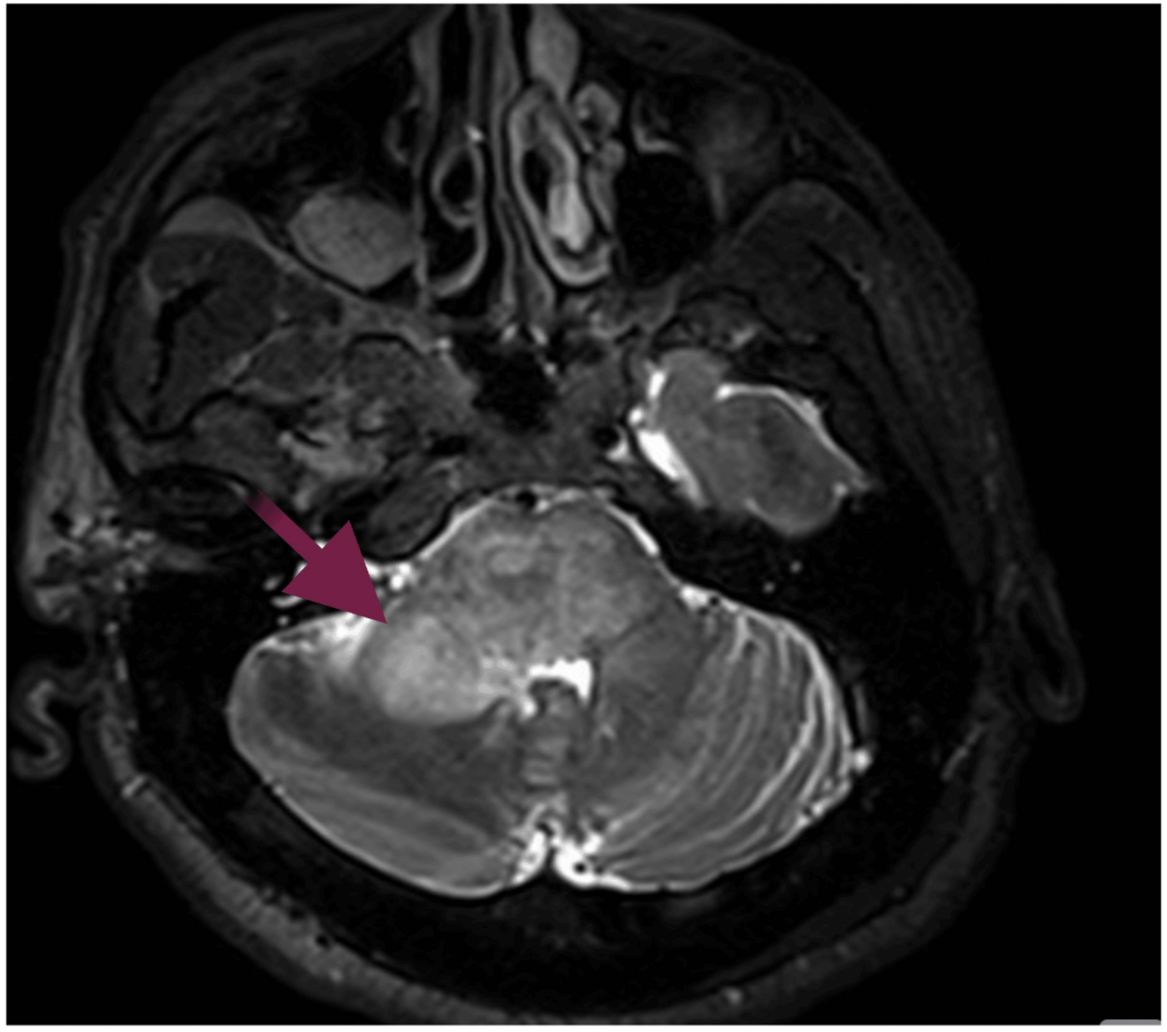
MRI of the brain MRI of the brain demonstrated an enhancing intra-axial mass (red arrow) with central hemorrhagic necrosis in the right pons. This critical finding raised concern for metastasis versus primary high-grade glioma, prompting urgent systemic malignancy workup MRI: magnetic resonance imaging
